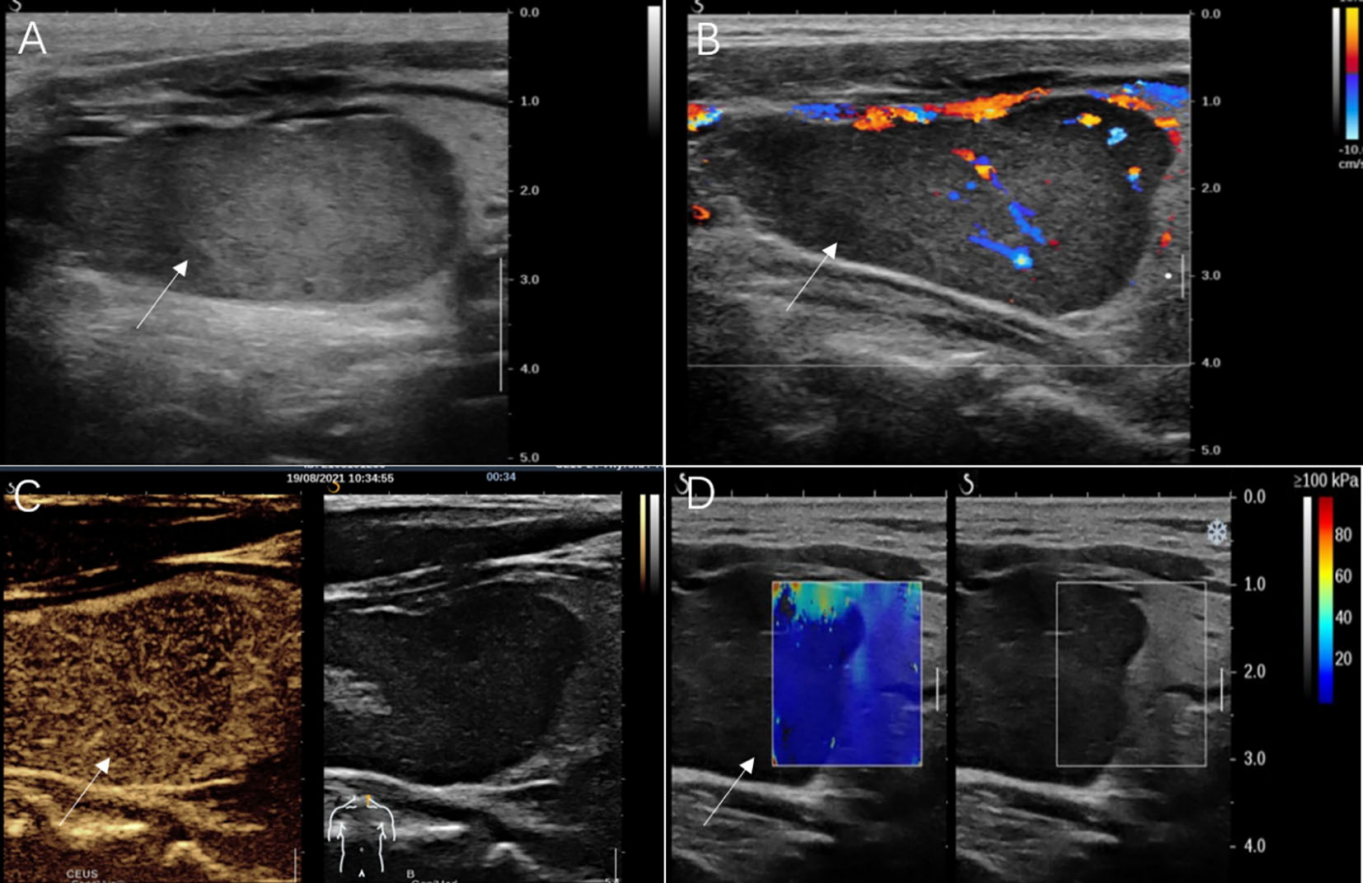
Ultrasonography images of adult-type rhabdomyoma of the thyroid. (A) Conventional gray-scale sonography revealed a solid and hypoechoic thyroid nodule (arrow) in the left lobe. (B) Color Doppler flow imaging showed a relatively rich blood flow signal inside this nodule (arrow). (C) Contrast-enhanced ultrasound image showed hypoenhancement of the nodule (arrow) in the arterial phase. (D) Shear wave elastography showed a lower stiffness of the nodule (arrow) compared with that of the thyroid.
